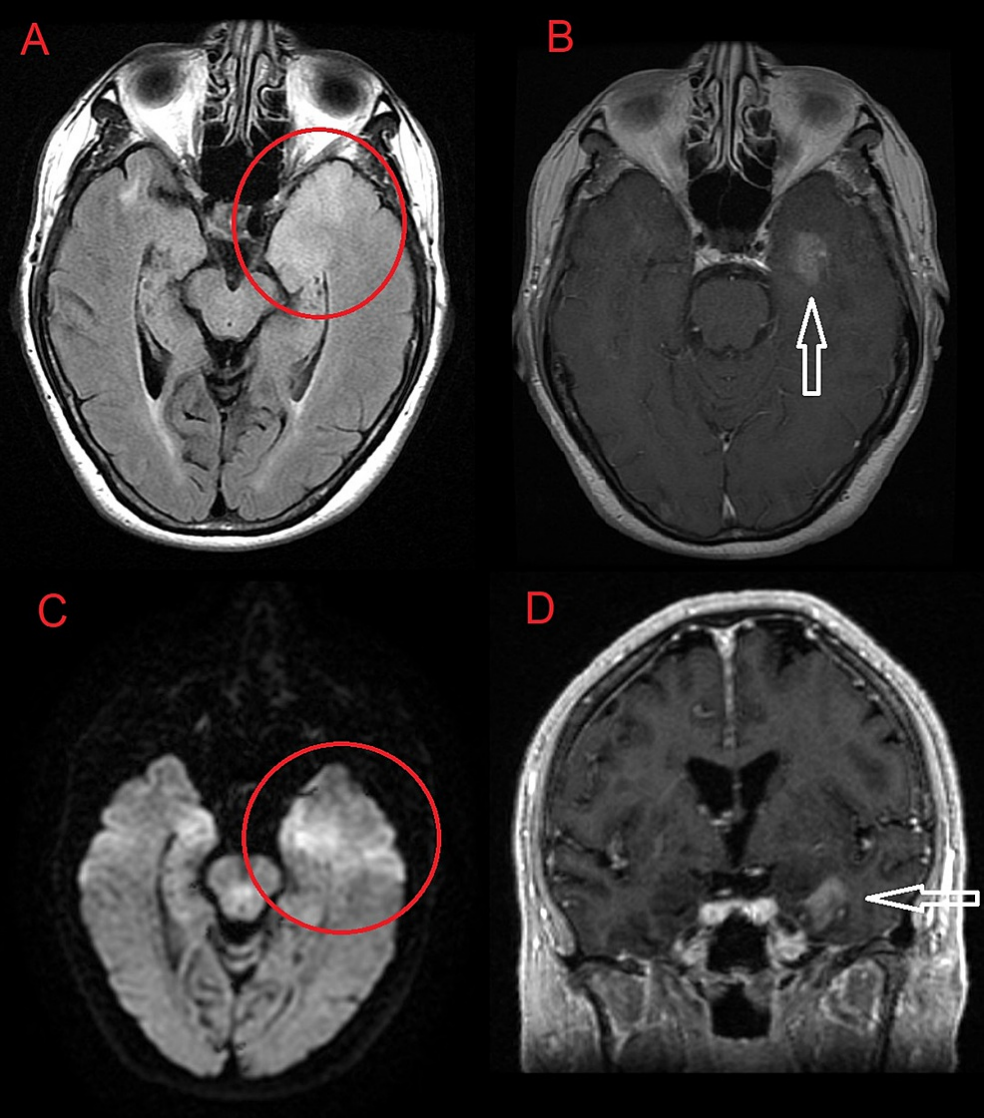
MRI of the brain. Axial FLAIR (A), T1-weighted with contrast (B), diffusion-weighted (C), and coronal T1-weighted with contrast (D) imaging of the brain demonstrate a 1.8 × 1.6 cm area of cortical and subcortical FLAIR hyperintensity (A) with a small internal focus of mild diffusion restriction (C) and amorphous enhancement in the anteromedial aspect of the left temporal lobe involving the uncus, amygdala, and hippocampal head (B, D). FLAIR: fluid-attenuated inversion recovery; MRI: magnetic resonance imaging
